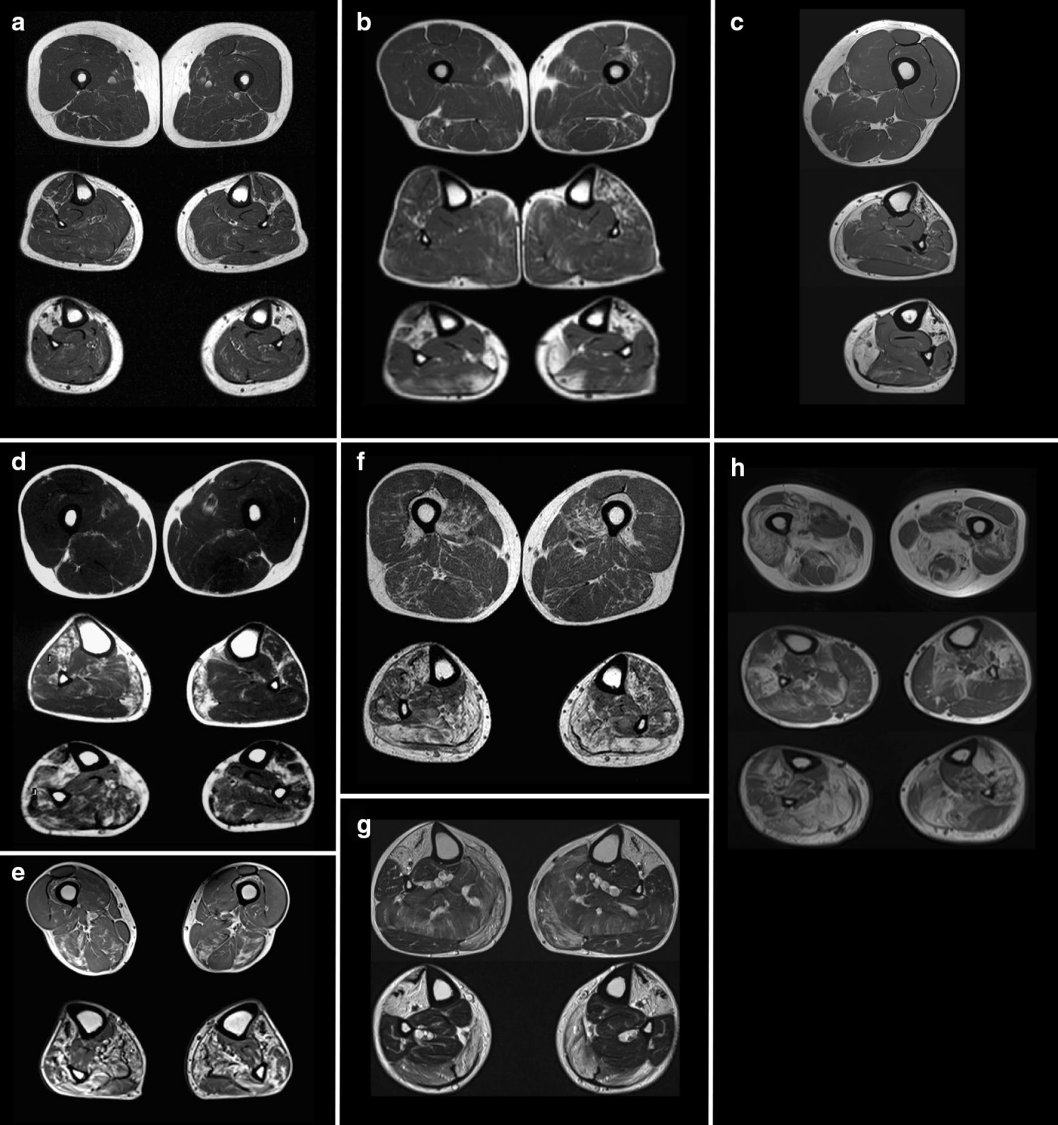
Magnetic resonance imaging (MRI) T1 sequences of patients with SMPX distal myopathy. a Patient F1 II.1 at 57 yrs showing normal thighs but fatty degeneration in lower legs: anterior compartment and medial gastrocnemius muscles. b F2 II.2 at 61 yrs showing minor degenerative change in thigh muscles semimembranosus, biceps femoris and left vastus intermedius; fatty degenerative changes in the anterior compartment muscles more on the left of proximal lower leg and fatty replacement of anterior compartment and part of the soleus muscles in the distal lower legs. c F3 II.1 at 58 yrs (left lower limb) with normal thigh and severe fatty degeneration in lower leg anterior compartment, medial gastrocnemius and medial part of distal soleus. d F6 II.1 at 52 yrs showing normal thigh and fatty degenerative changes in the anterior compartment (more on the right) and medial gastrocnemius muscles of proximal lower leg, and anterior compartment with soleus muscles in the distal lower legs. e F6 II.1 at 60 yrs with early degenerative changes in biceps femoris and semimembranosus in the thigh and more fatty degeneration of anterolateral compartments and of medial gastrocnemius and soleus in the lower legs. f F7 II.1 at 56 yrs shows milder fatty degeneration in vastus intermedius and medialis, semimembranosus and biceps femoris on the thigh, and in both posterior and anterior compartments on the lower legs, less in the lateral peroneal muscles. g F8 III.1 at 58 yrs shows lower legs with severe fatty replacement of anterior compartments and medial heads of gastrocnemius and milder changes in the soleus. h F9 II.1 at 76 yrs showing pronounced fatty degeneration in thighs: more in hamstrings than the lateral and intermediate vastus of the quadriceps, severe changes in distal lower leg anterior compartment and soleus muscles and peroneals more on the right
